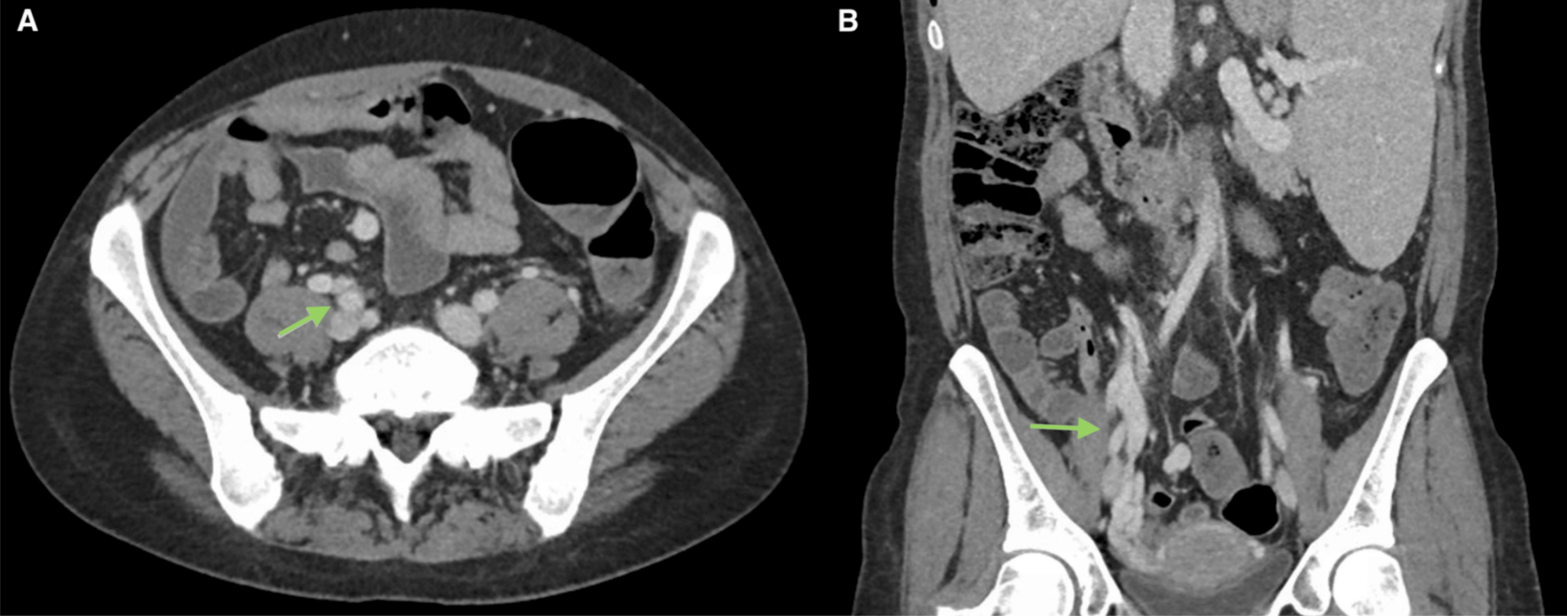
(A) Axial view of CT angiography (CTA) showing distal ileal varices. (B) Coronal view of CTA showing ileal varices and venous congestion of the uterus due to portosystemic shunting via the superior mesenteric vein to the right gonadal vein.
